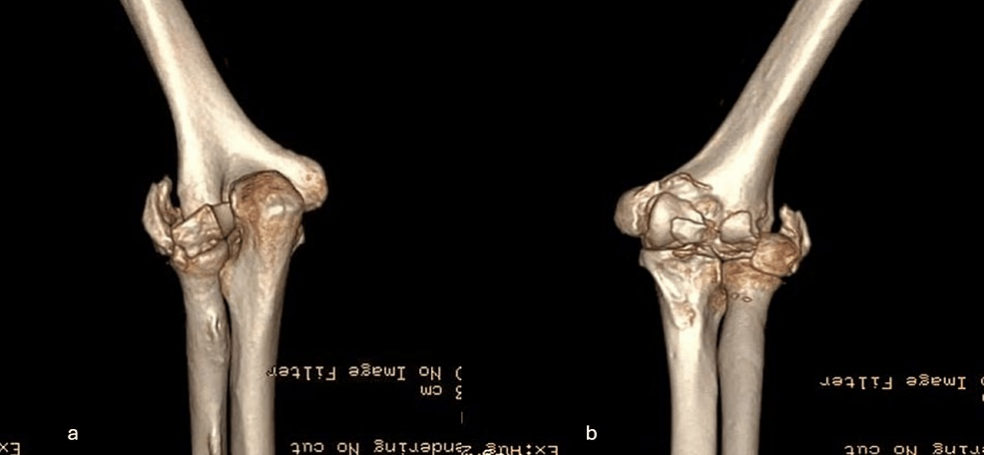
Three-dimensional CT scan of the elbow before the procedure showing the non-union of the lateral condyle and the valgus deformity of the elbow: (a) posterior view and (b) anterior view of the joint.
